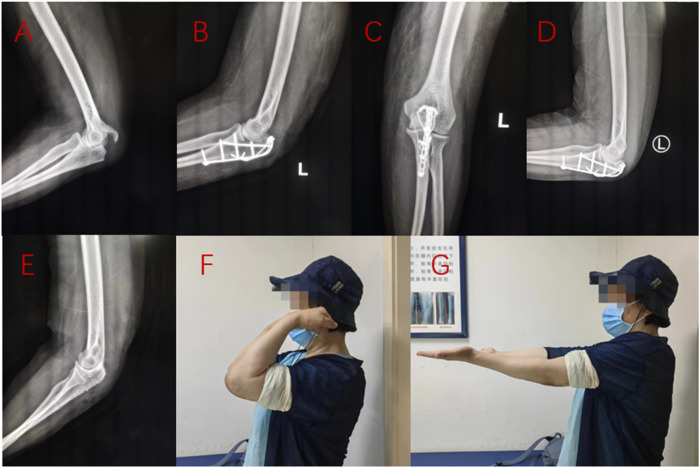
A 61-year-old female patient suffered from left olecranon fracture due to fall injury. Preoperative X-ray examination (A), X-ray examination (B, C) 1 day after anchor fusion plate fixation, X-ray examination (D) 3 months after operation, X-ray examination (E) 1 year after internal fixation removal, and elbow function (F, G) 15 months after operation were performed.
